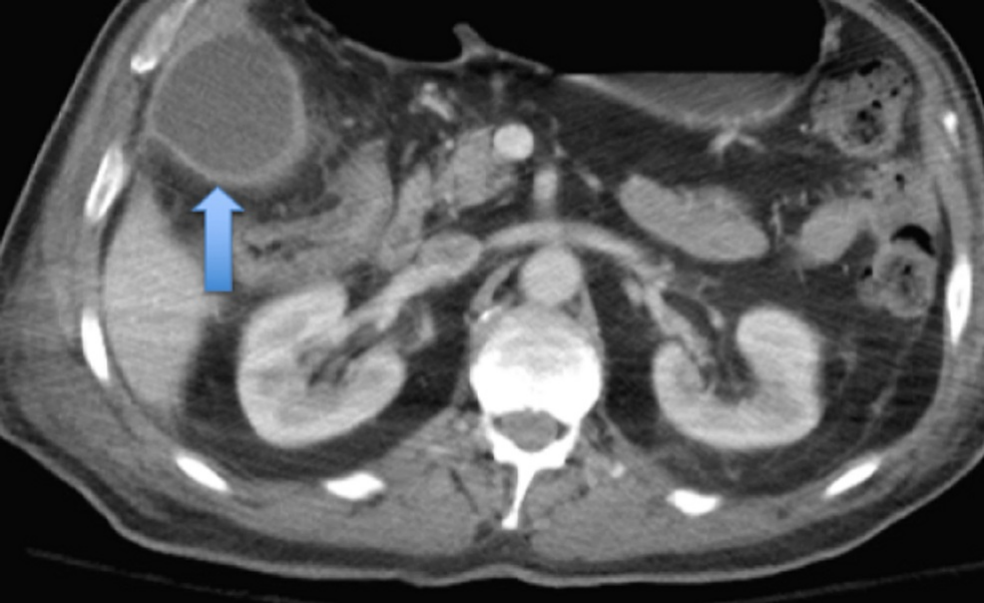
Computed tomography (CT) abdomen and pelvis with contrast (axial view). Dilated and inflamed gallbladder consistent with acute cholecystitis. Gallbladder wall thickening (blue arrow).
